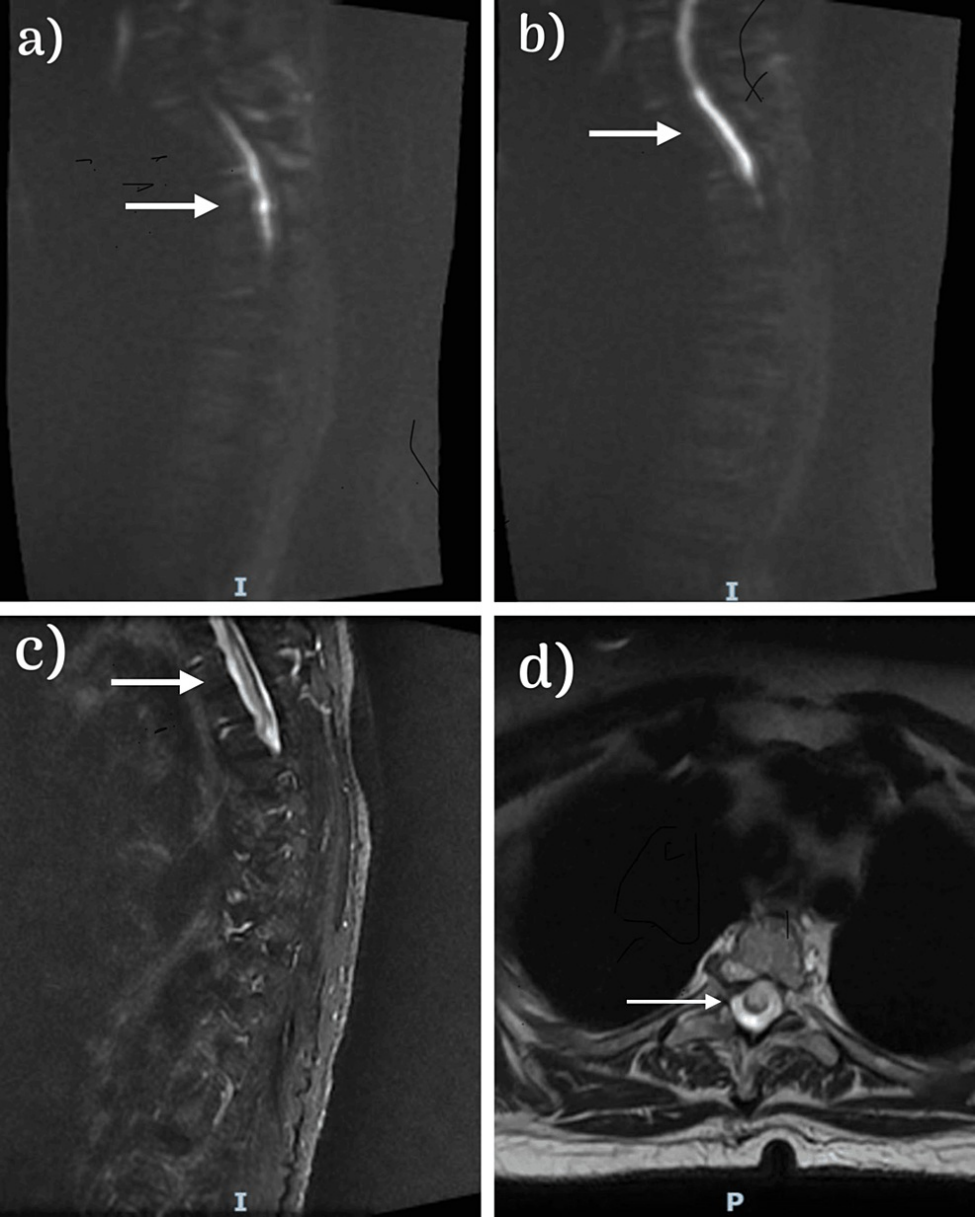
Magnetic resonance imaging of the cervical and thoracic spines (a, b) Restricted diffusion on the diffusion-weighted imaging in the spine from T1 to T7. (c, d) A long segment of intramedullary hyperintensity observed in the T2 image. This hyperintensity is mainly located ventrally and to a lesser extent centrally at the level T1-T7.
